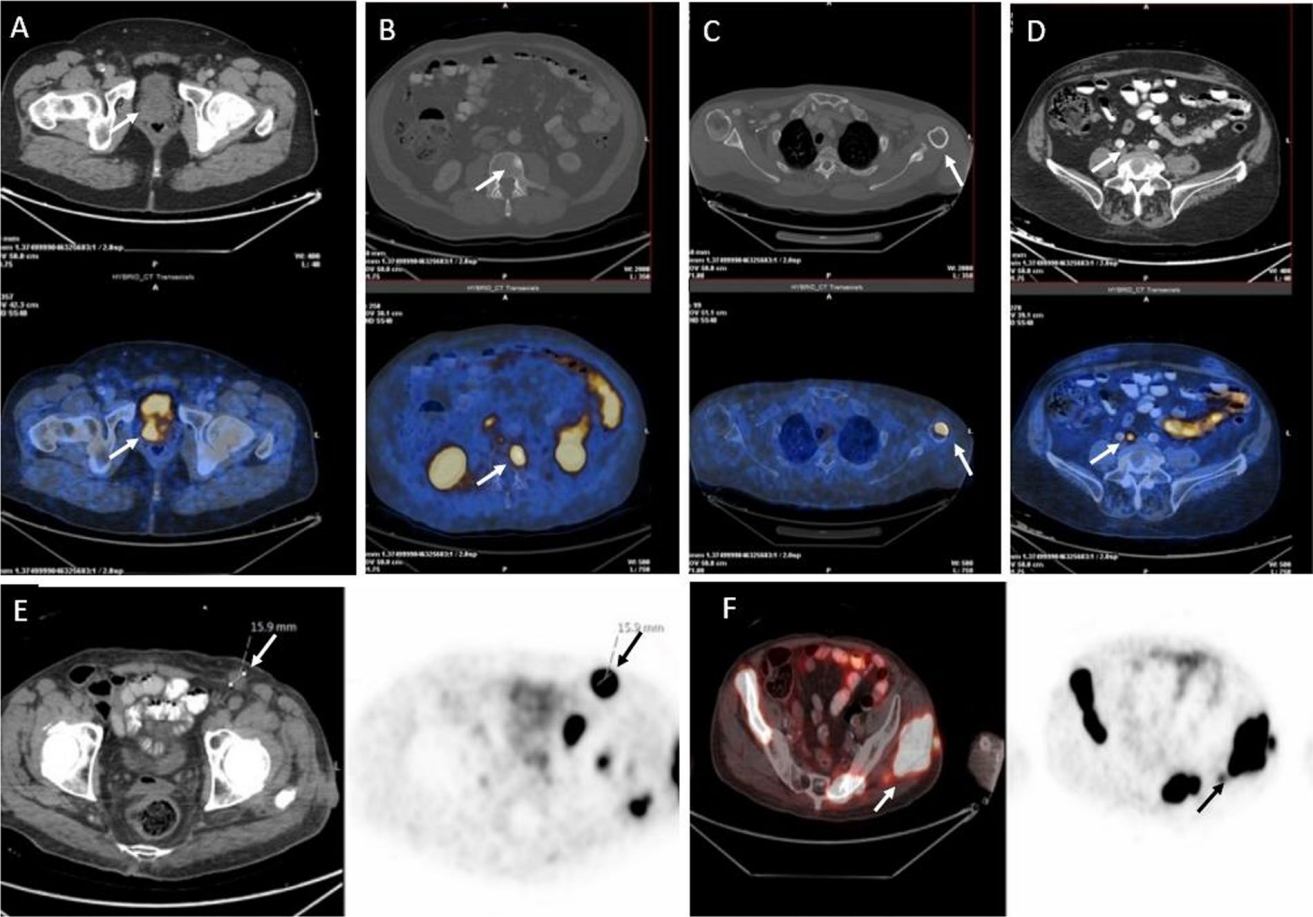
Examples of different sites showing pathological 68Ga-isoPROtrace-11 uptake: (A) Prostate tumour, (B) Bone metastasis with a CT finding, (C) Bone metastasis with no finding on CT, (D) Small (up to 15 mm) lymph node metastasis, (E) Enlarged (more than 15 mm) lymph node metastasis, (F) Soft tissue metastasis (see arrows).
